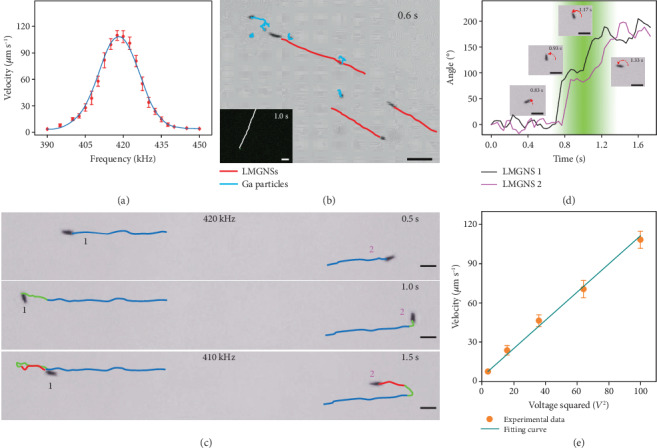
The acoustically propelled motion of the LMGNSs. (a) The relationship between the motion velocity of LMGNSs and frequency of acoustic field at 10 V. (b) Time-lapse image of a LMGNS at an applied voltage of 10 V and an ultrasound frequency of 420 kHz. Inset is fluorescent time-lapse image of a LMGNS under same condition. (c) The time-lapse images of frequency determined direction control of LMGNSs. (d) The direction changes of LMGNSs during frequency changing. The inset images show the LMGNS 2 at 0.83, 0.93, 1.17, and 1.33 s. (e) The relationship between motion velocity LMGNSs and the applied voltage of acoustic field. Scale bars, 20 *μ*m.
